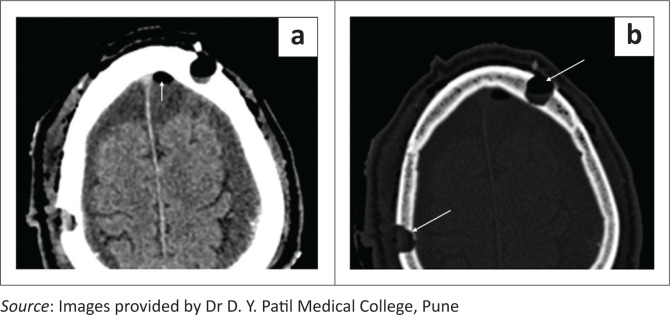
Axial soft tissue (a) and bone window (b) CT head images showing burr holes, seen as rounded well defined defects in the skull bones in the left frontal and right parietal regions (long white arrows). Note the small postoperative pneumocephalus in the left frontal region (a, short white arrow).
